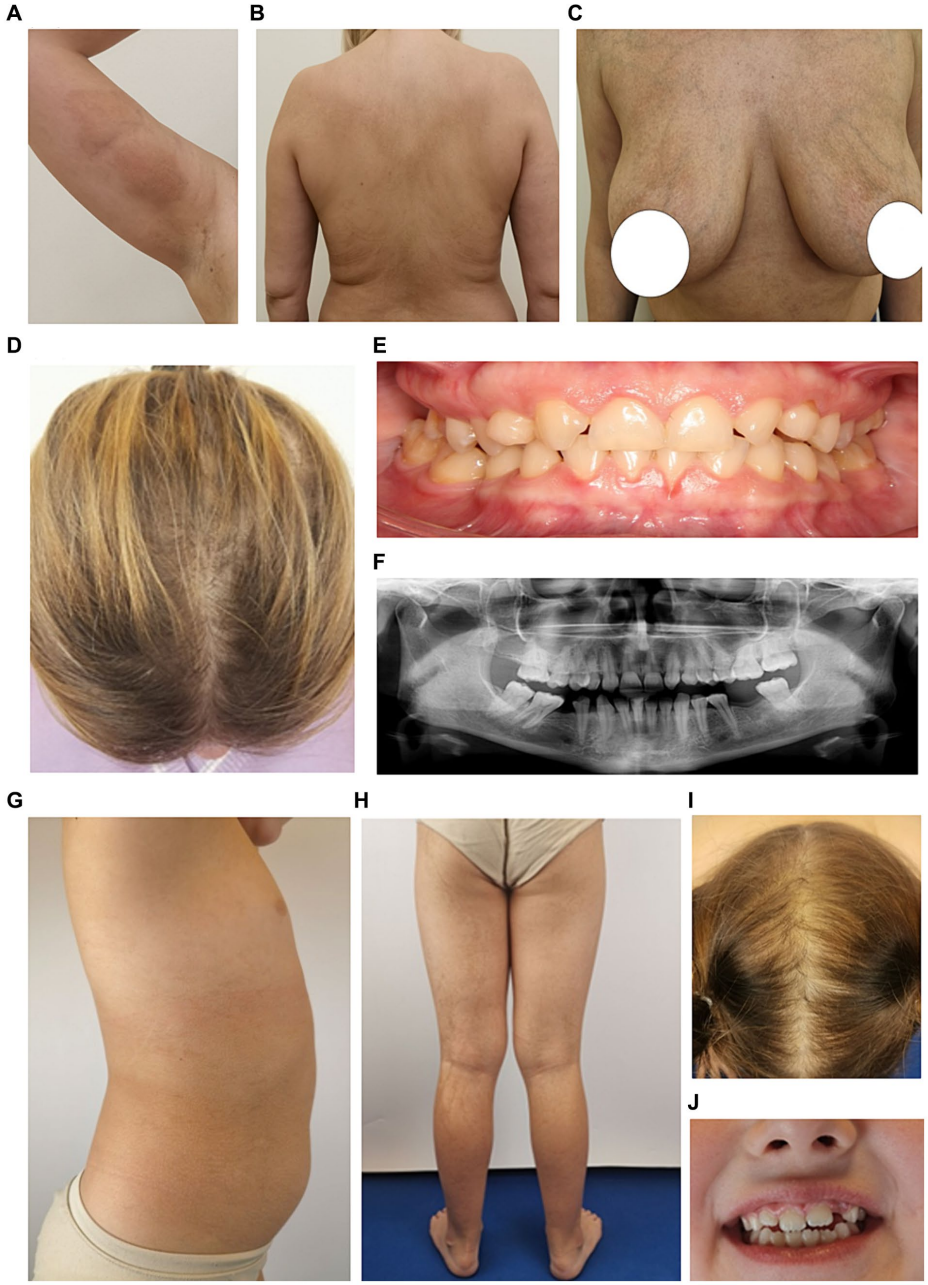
Phenotypic characteristics of the proband’s mother M (A–F) and sister S1 (G–J). (A–C) Areas of irregular pigmentation in M. (D) Parietal zone with hair thinning. (E) Dental malocclusion (adentia of both upper lateral insicors and also two molars on the lower jaw are missing). (F) Orthopantomogram shows anomalies in the positioning of individual teeth, adentia of both maxillary lateral insicors and two molars on the lower jaw. (G,H) Areas of hyperpigmentation on the trunk and legs in S1. (I) Parietal zone with sparse hair growth. (J) Mild dental malformations.
